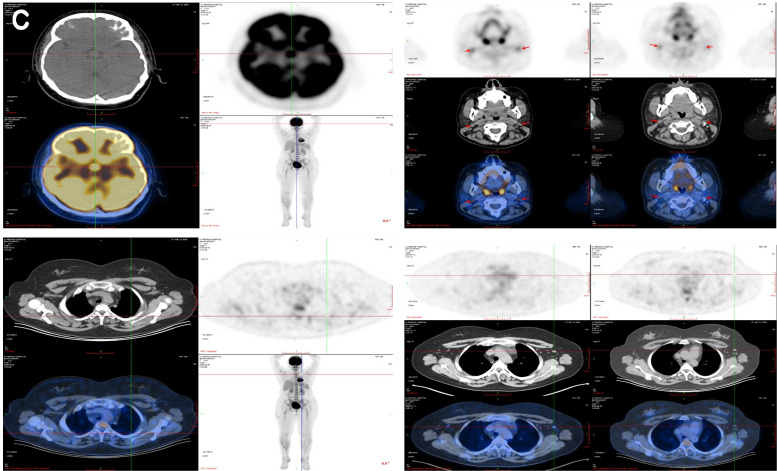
Whole-body PET–CT evaluation after 6 cycles of chemotherapy showing partial response with decreased size and metabolic activity of residual lesions.
